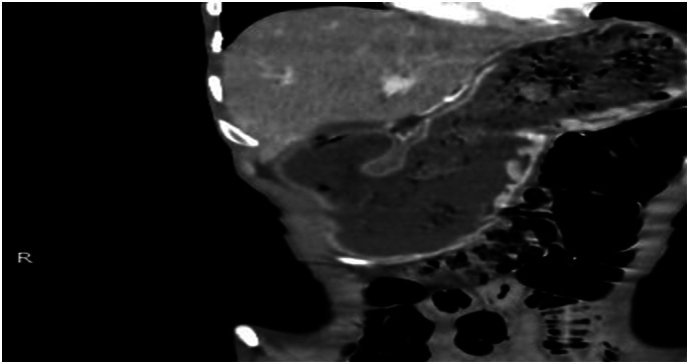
Abdominal CT scan in coronal section shows distended fluid filled stomach with outlet smooth tapering and multiple enlarged per pyloric lymph nodes.
